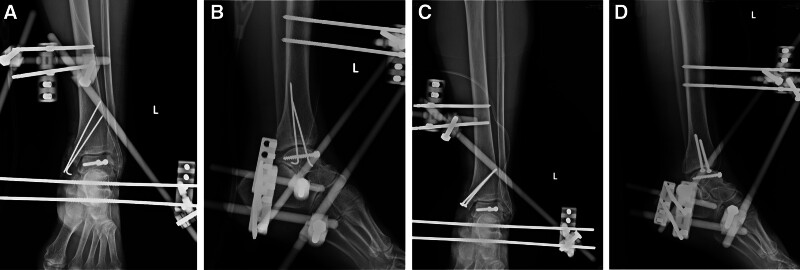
Postoperative follow-up radiograph after the initial operation showing the talus after reimplantation and external fixation (A, B) and after the second stage operation (C, D).
